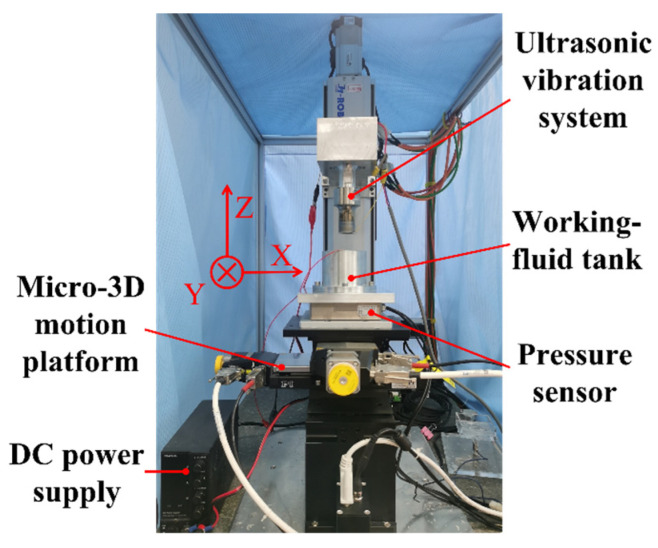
Physical map of the template electrophoretically assisted micro-ultrasonic machining device.
